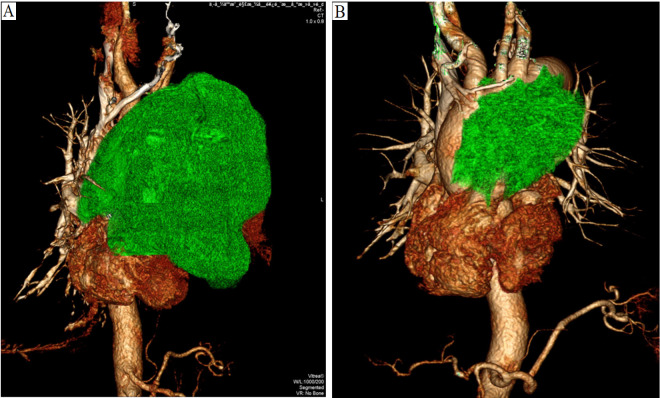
(A). Enhanced CT angiography of the three-dimensional reconstruction reveals that the upper margin of the tumor extends beyond the aortic arch, while the lower margin reaches the inferior border of the heart, compressing the aortic arch, thoracic aorta, left pulmonary artery, and heart (2023-01-10). (B). After a year of treatment, the tumor showed significant shrinkage (2024-02-23).
